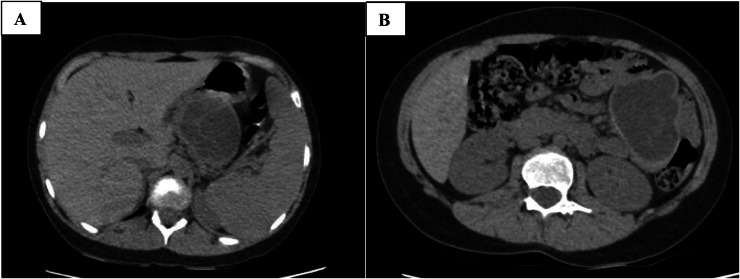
In limited view of abdomen of the high-resolution computed tomography images, a multiloculated cystic structure with hyperdense wall and septa is seen (A and B).
